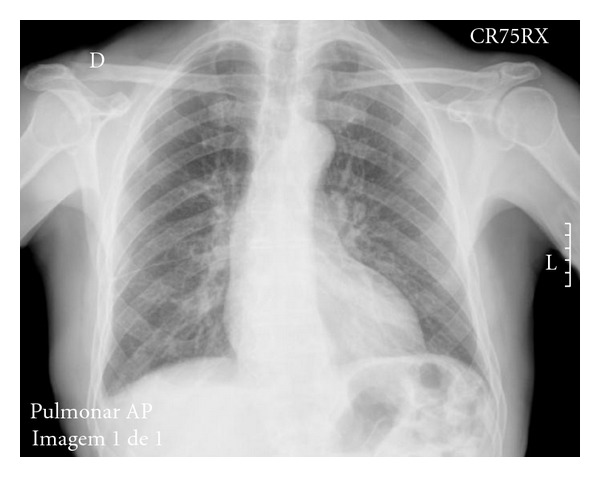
Chest X-ray one month after suspending the bromocriptine.
